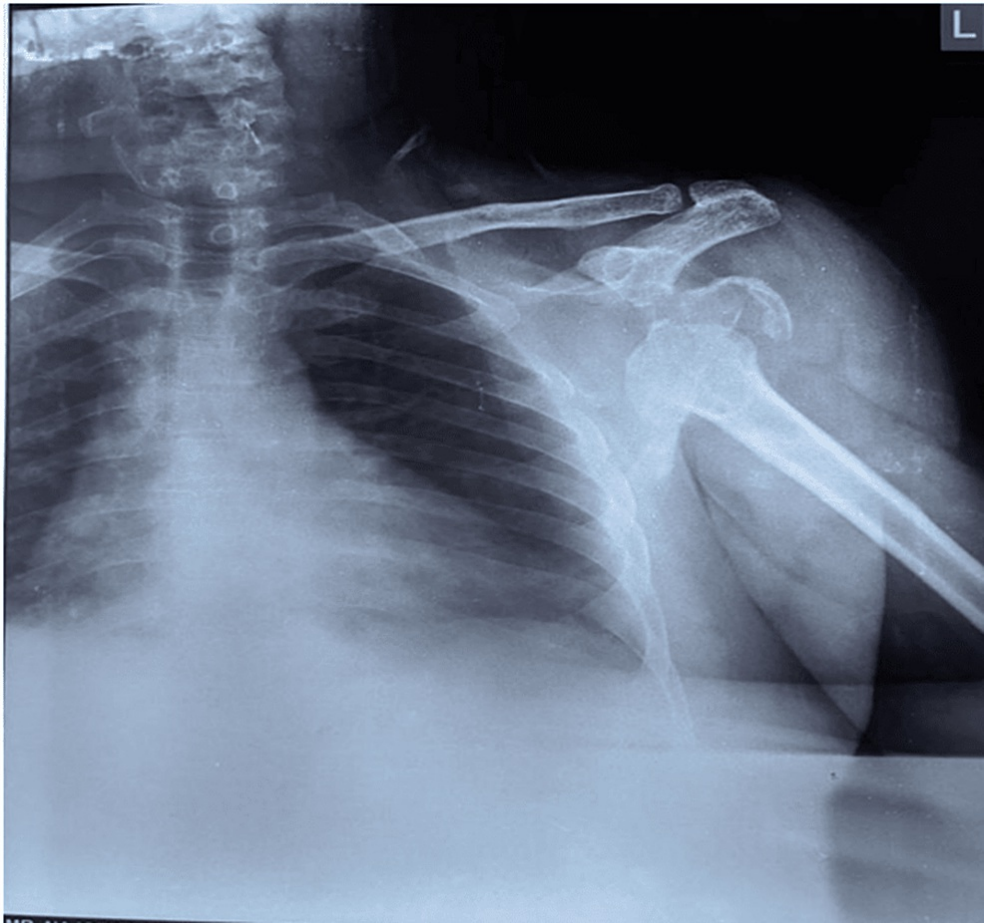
X-ray showing dislocation and fracture of the left shoulder joint.
